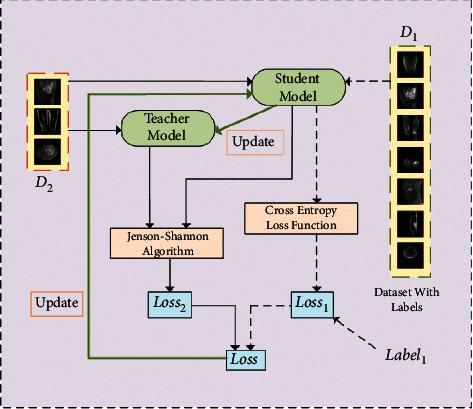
Optimization process of the osteosarcoma MRI image dataset based on mean-teacher semisupervised algorithm.
